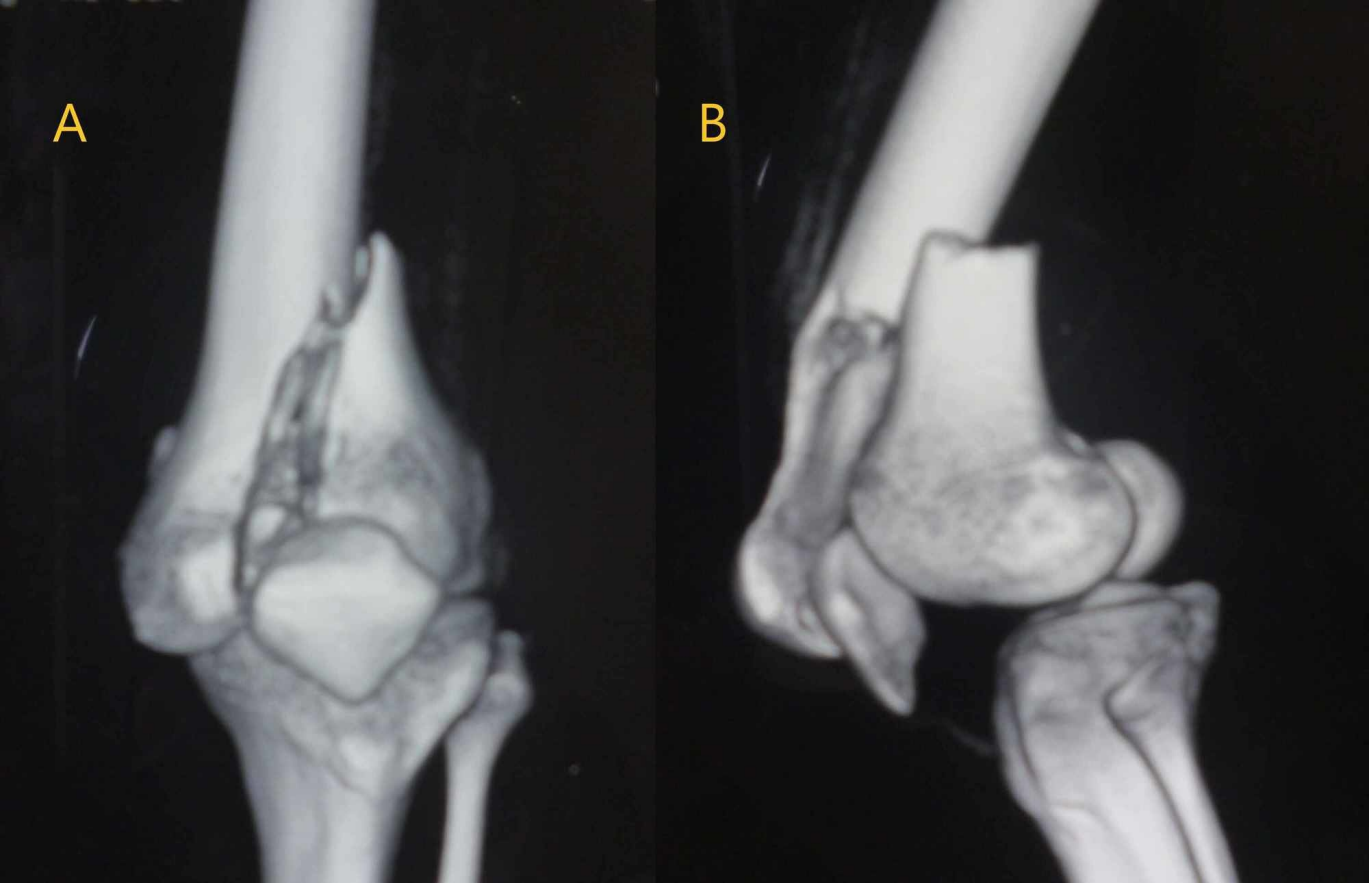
Three-dimensional reconstruction of the CT scan A: anteroposterior view; B: lateral view. The images show the sagittal intercondylar separation of the distal femur and the proximal shaft with part of the articular surface (coronal fracture) in continuation with the intact metaphysis CT: computed tomography
